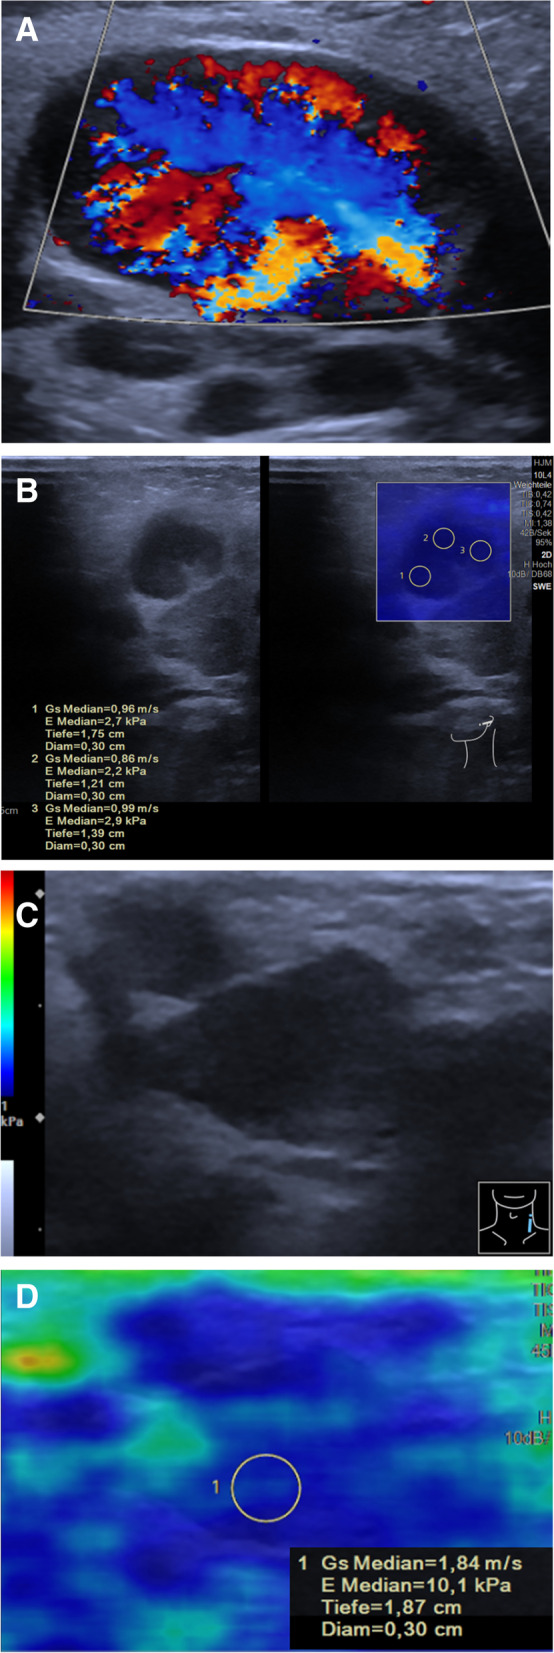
Ultrasound elastography in peripheral cervical lymph nodes. A Color Doppler image of a lymph node near the mandible in a 2-year-old male with periapical osteitis caused by caries profunda. There is strong hypervascularization without swelling (Sequoia, Siemens, L14-5 probe). B Same case with SWE showing shear wave velocity < 1 m/s (Sequoia, Siemens, L10-4 probe). C Color Doppler imaging of a new cervical lymph node in a 14-year-old male with history of Burkitt lymphoma. There was no vascularization detectable (Sequoia, Siemens, L14-5 probe). D Same lymph node with SWE showing shear wave velocity of 1.84 m/s corresponding with increased stiffness. Imaging and elastography are suspicious for relapse (Sequoia, Siemens, L10-4 probe)
